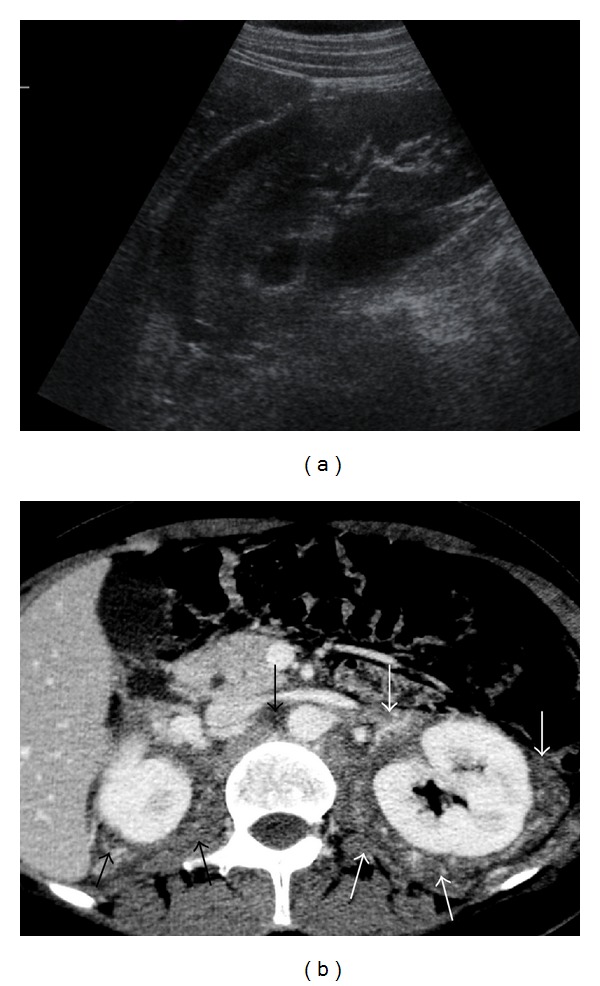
NHL. (a) Right kidney sonogram shows hypoechoic perinephric mass (arrows). (b) Axial contrast-enhanced CT scan shows a large soft-tissue mass (arrows) infiltrating the retroperitoneum and extending into the perinephric space bilaterally.
